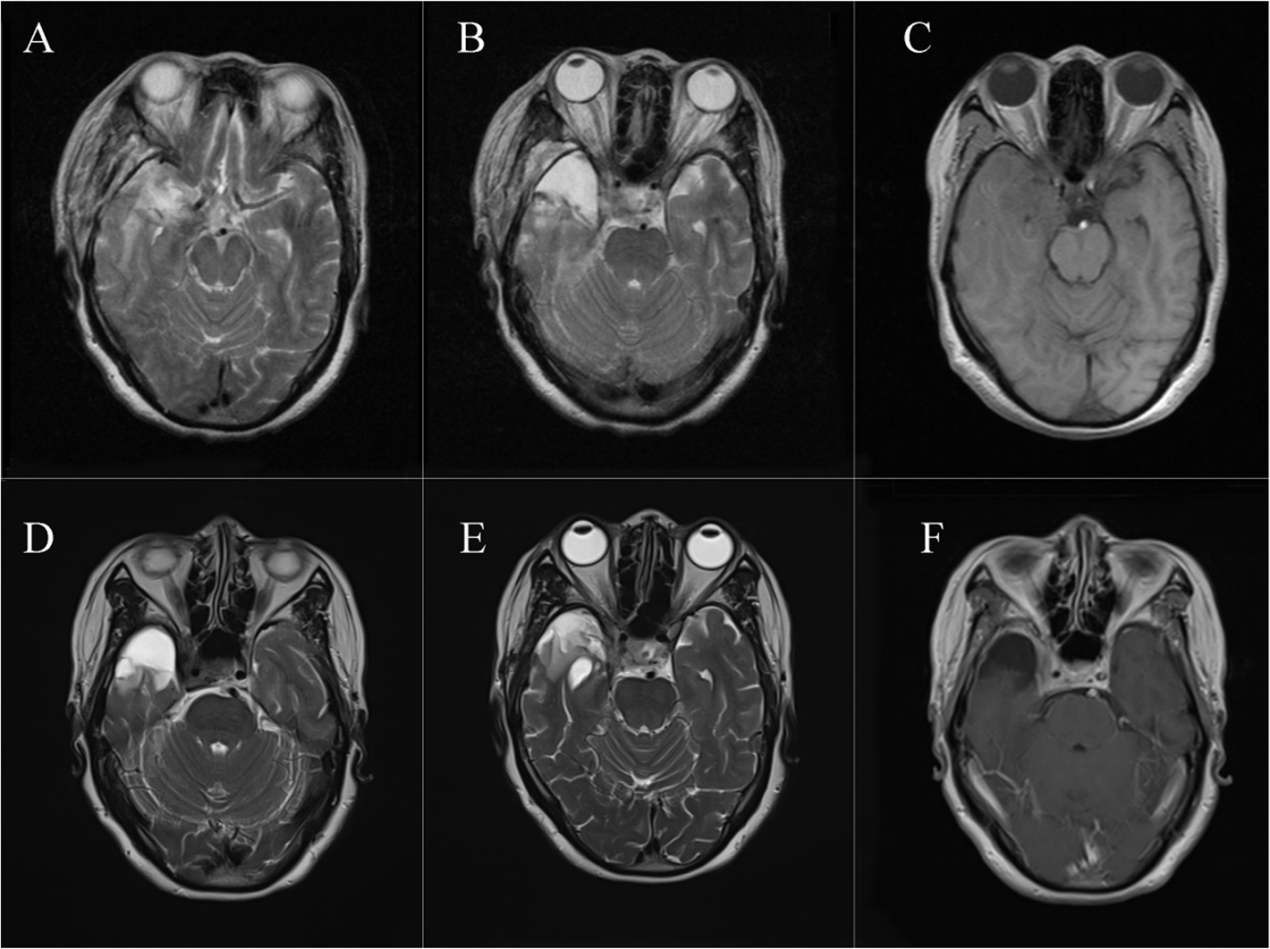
MR images after surgery: MR images 15 days after surgery (a, b, c). MR images 6 months after surgery (d, e, f). The new lesion was completely removed, and the CSM achieved partial removal. The temporal lobe edema area has decreased remarkably on the T2WI images
